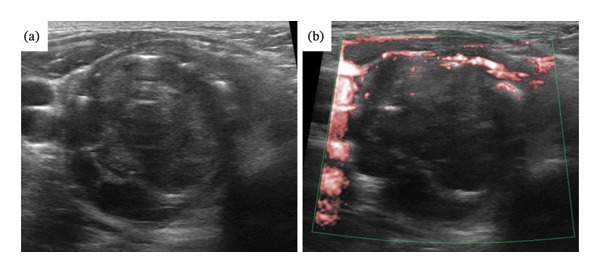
Ultrasonography showed a 4.5‐cm tumor in the right lobe of the thyroid gland. (a) The internal echoes were heterogenous. Lymph node metastasis was observed below the thyroid tumor. (b) The blood flow signals inside the tumor were poor.
